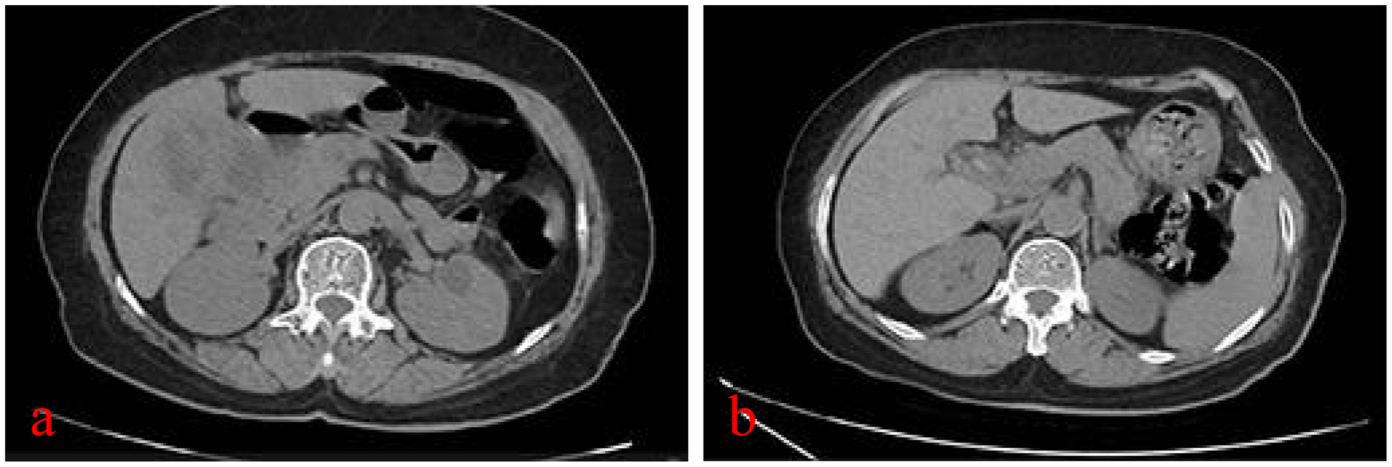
CT plain scan of the abdomen at the time of the patient's two admissions: (a) first admission, severe inflammatory reaction in and around the gallbladder; and (b) second admission, inflammatory reaction in and around the gallbladder has subsided.
